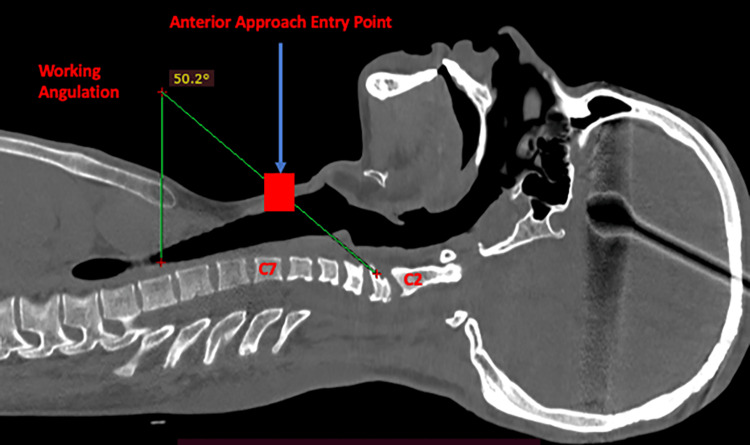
CT sagittal view showing difficulties encountered during an anterior approach with a working angulation of 50.2 degrees.
